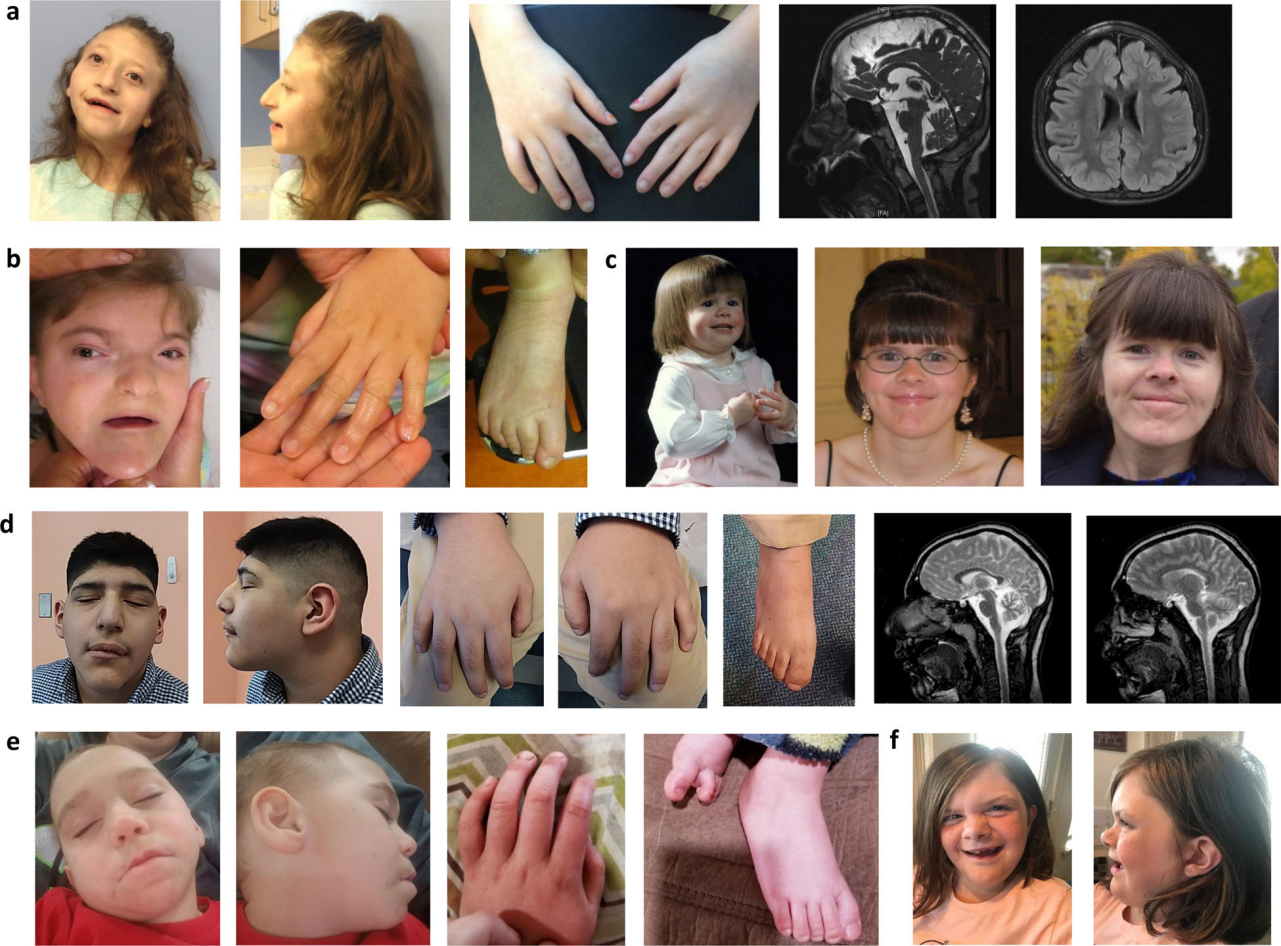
Images showing dysmorphic profiles involving facial features, hands and/or feet, and brain MRI images for available individuals. a Individual 1, b Individual 5, c Individual 6 (left to right: 20 months, 21 years, 33 years), d Individual 8, e Individual 9, and f Individual 10. Written informed consent form has been obtained from families for the publication of facial photos, and brain MRI images when applicable.
